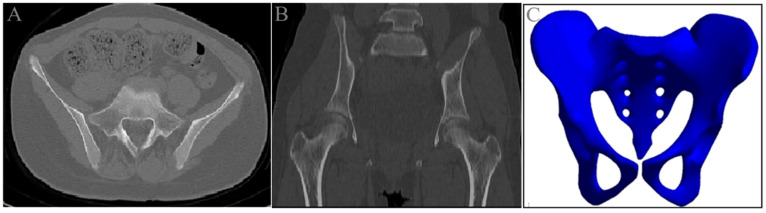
Three-dimensional reconstruction of the pelvis. (A) Pelvic cross-sectional CT scan; (B) pelvic coronal CT scan; (C) 3D reconstruction model of the normal pelvis.
